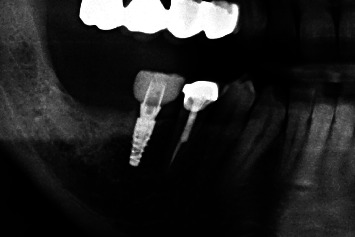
Regio #30-postoperative X-ray control (detail from panoramic tomogram).
